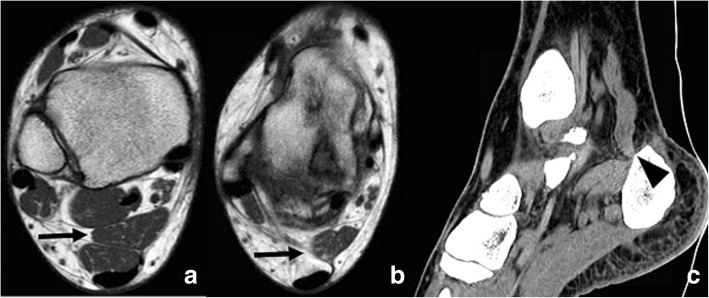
Accessory soleus. 36-year-old woman referred for follow-up of an osteochondral lesion. Incidental finding of an accessory soleus. Axial FSE T1 at different levels from (a) proximal to (b) distal demonstrates the muscle belly anterior to the soleus, superficial to the flexor retinaculum (black arrows). The tendon descends to insert in the medial aspect of the calcaneus. c Sagittal plane CT reconstruction with soft tissue algorithm in the same patient also allows visualisation of the muscle, extending to insert in the calcaneus (black arrowhead)
